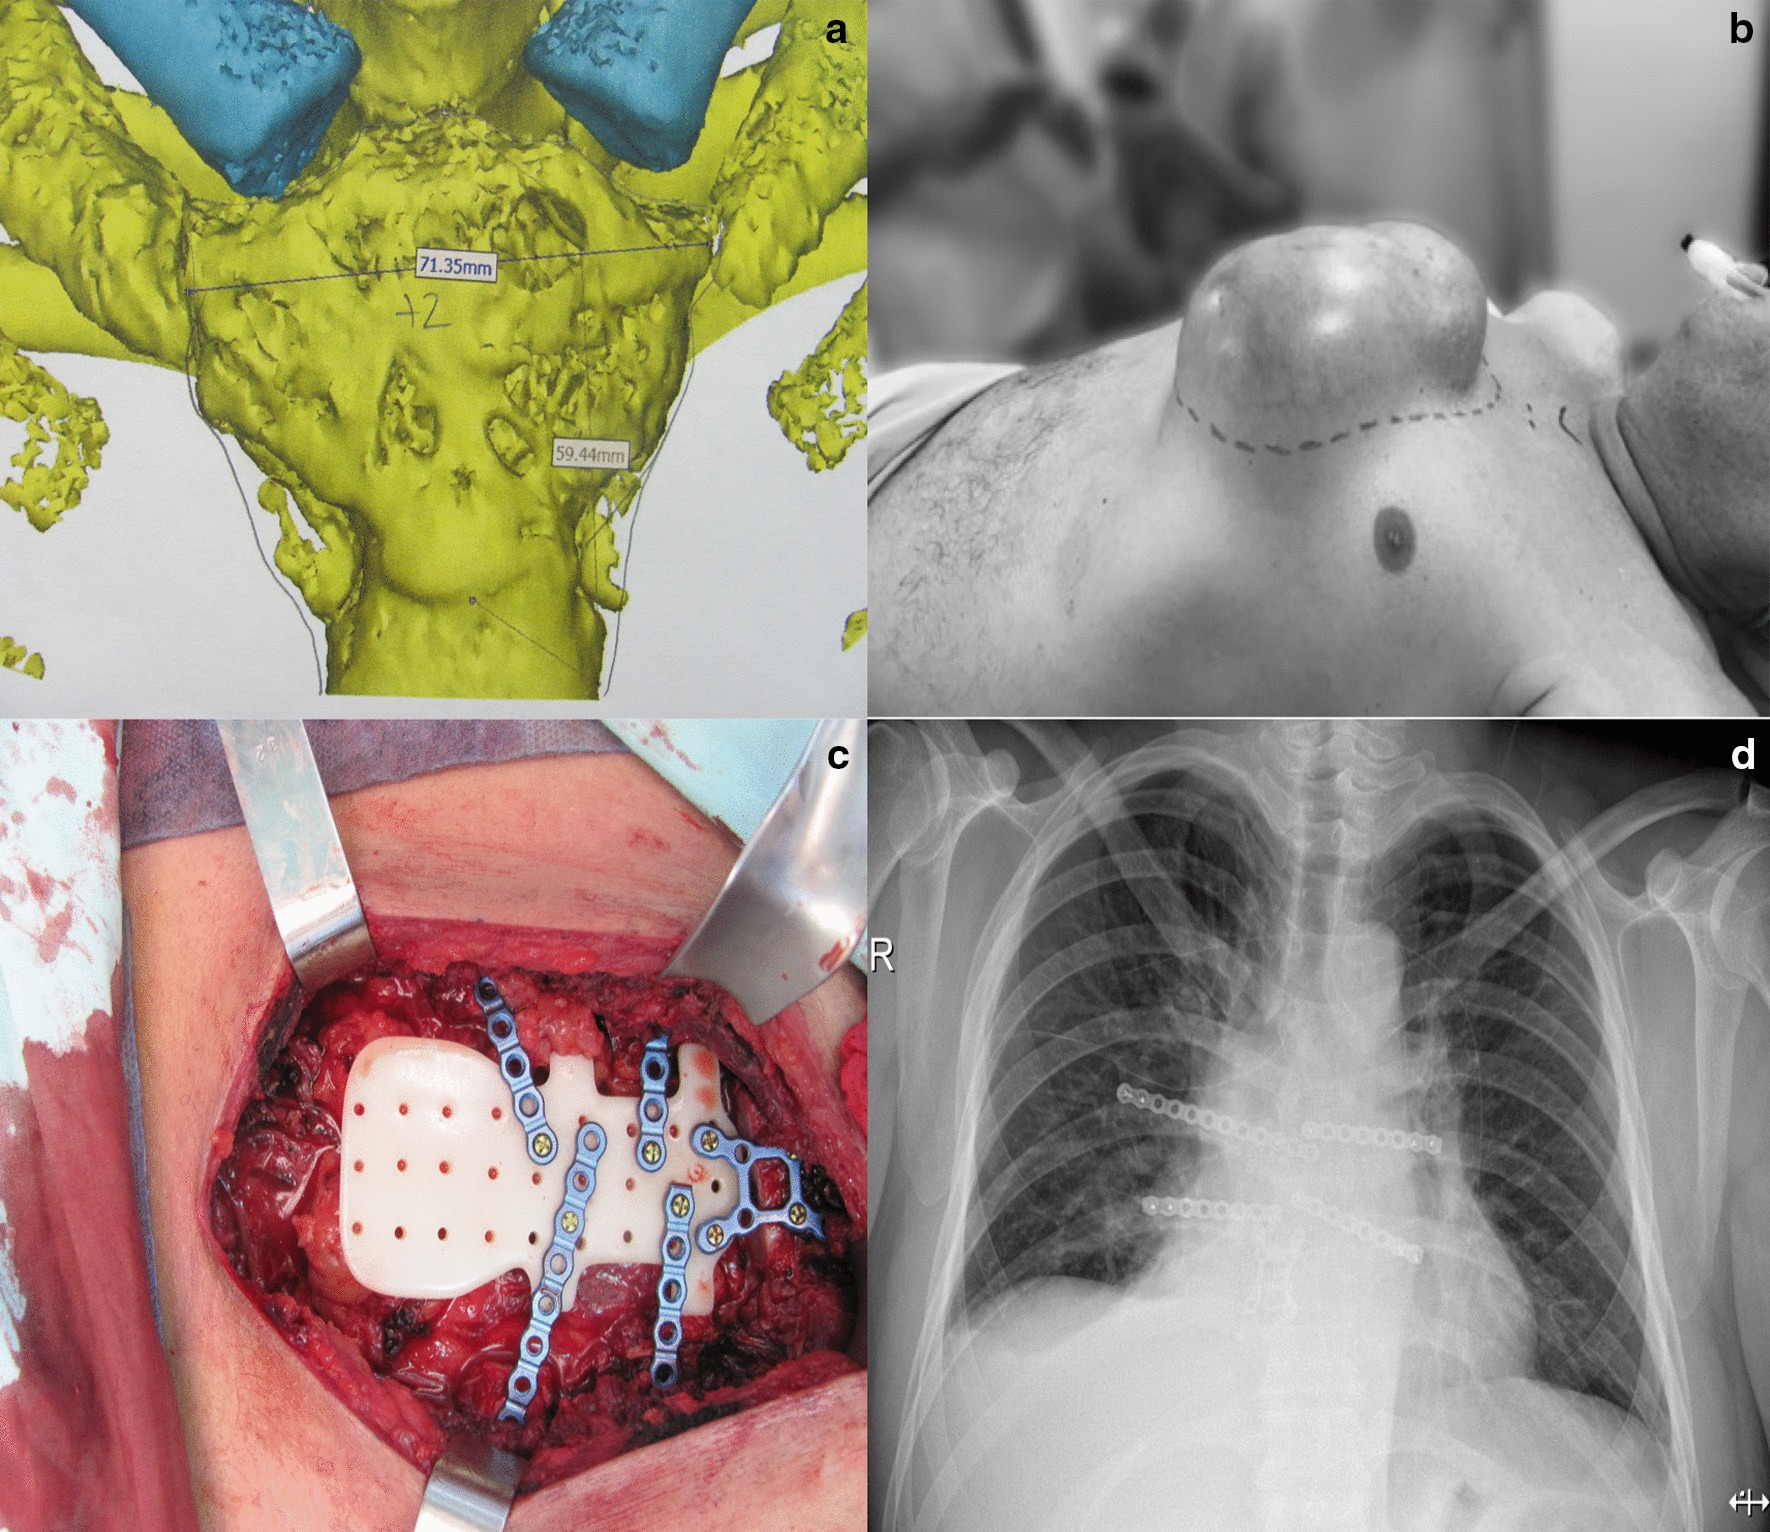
a 3D model to plan the sternal reconstruction. b Image of the mass protruding to the anterior wall of the thorax, over the sternal area, previous to surgery. c Surgical procedure with the installed prosthesis on the sternal space. d Post-surgical thoracic x-ray where the fixation elements of the prosthesis are evidenced in a good position
